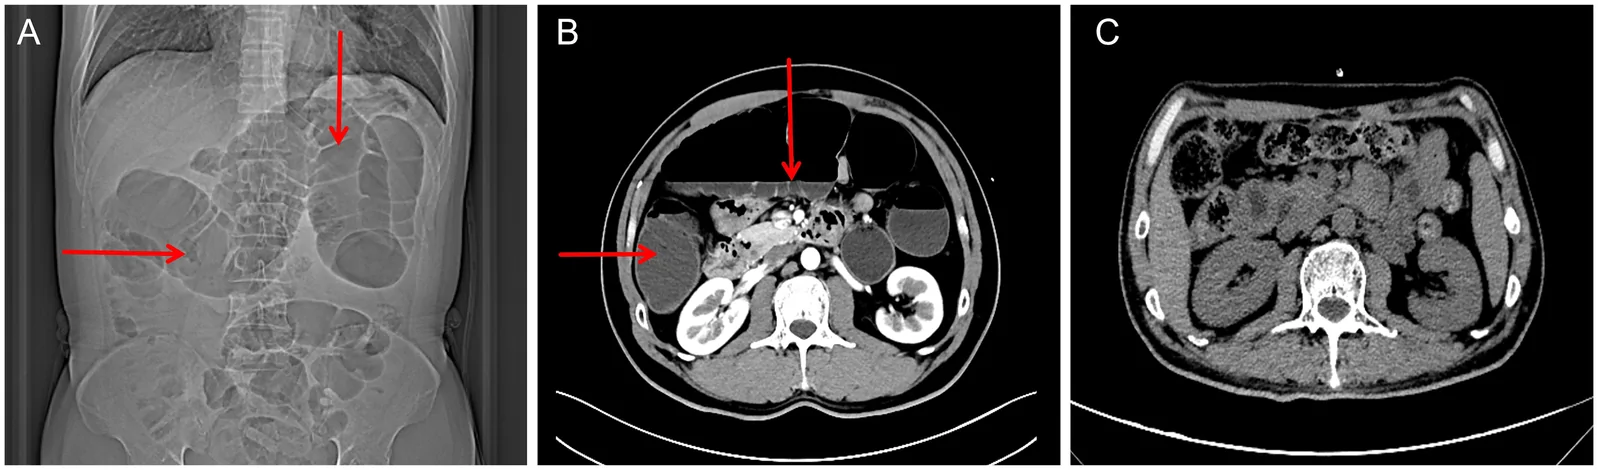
(A) (indicated by the arrow) shows dilated bowel loops on an abdominal X-ray. (B) (indicated by the arrow) shows diffusely dilated bowel loops with air-fluid levels on an axial contrast-enhanced abdominal CT. (C) Shows a follow-up axial abdominal CT obtained after chemotherapy and the subsequent relief of the patient’s bowel obstruction symptoms, demonstrating a marked reduction or disappearance of the dilated bowel loops and air-fluid levels compared to the pre-chemotherapy findings.
